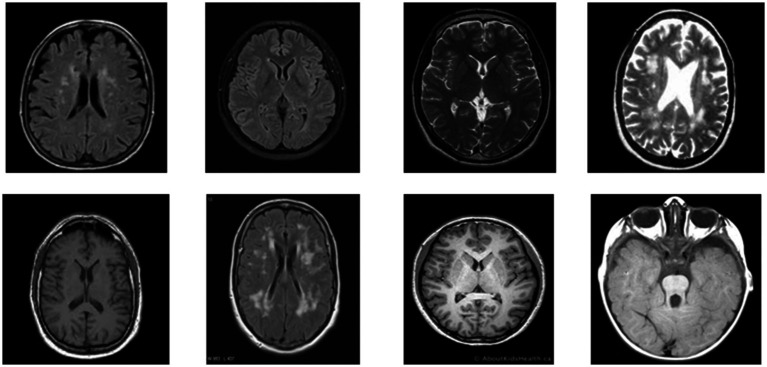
Visualization of healthy MRI scan.
